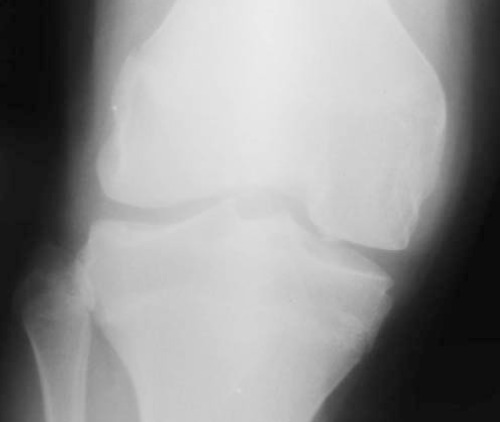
X-ray of the knee of the patient in Figure 2 shows narrowing inthe tibiofemoral joint space medially, increased subchondral sclerosis in thejoint spaces and minimal cortical irregularities in the medial condyle of the femur
